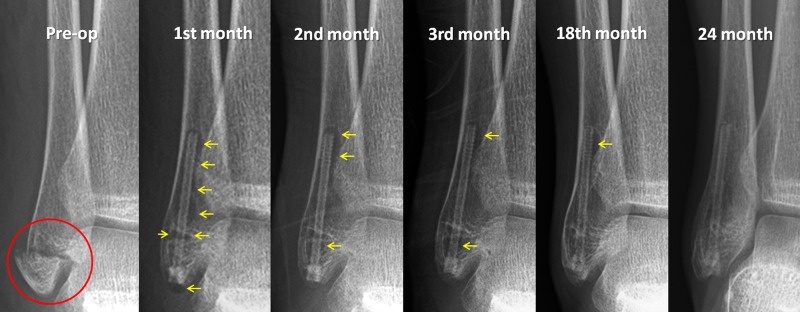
Serial radiographic examination Serial radiographic follow-up of the patient. Note the gradual reduction of the radiolucent zone around the screw at each subsequent follow-up examination up to 24 months. (The red circle shows the fracture and the yellow arrows show the radiolucent zones around the screw)
